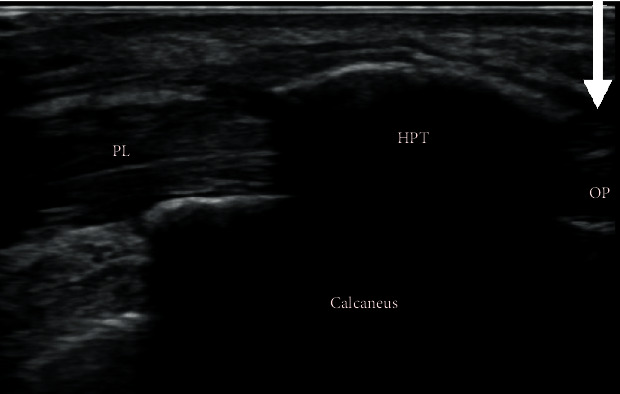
Dynamic ultrasonographic image confirmed hypertrophic peroneal tubercle and os peroneum causing impacts. Lidocaine injection was performed in between and os peroneum (white arrow). Abbreviation: PL: peroneus longus; HTP: hypertrophic peroneal tubercle; OP: os peroneum.
